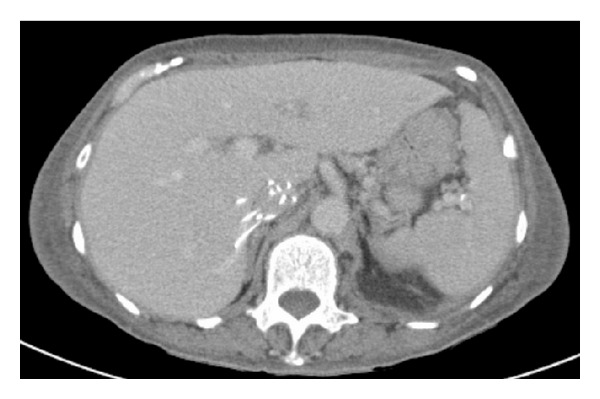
Preretrieval CT image showing obliquely oriented filter with perforation of IVC by the filter struts both medially and anteriorly.
